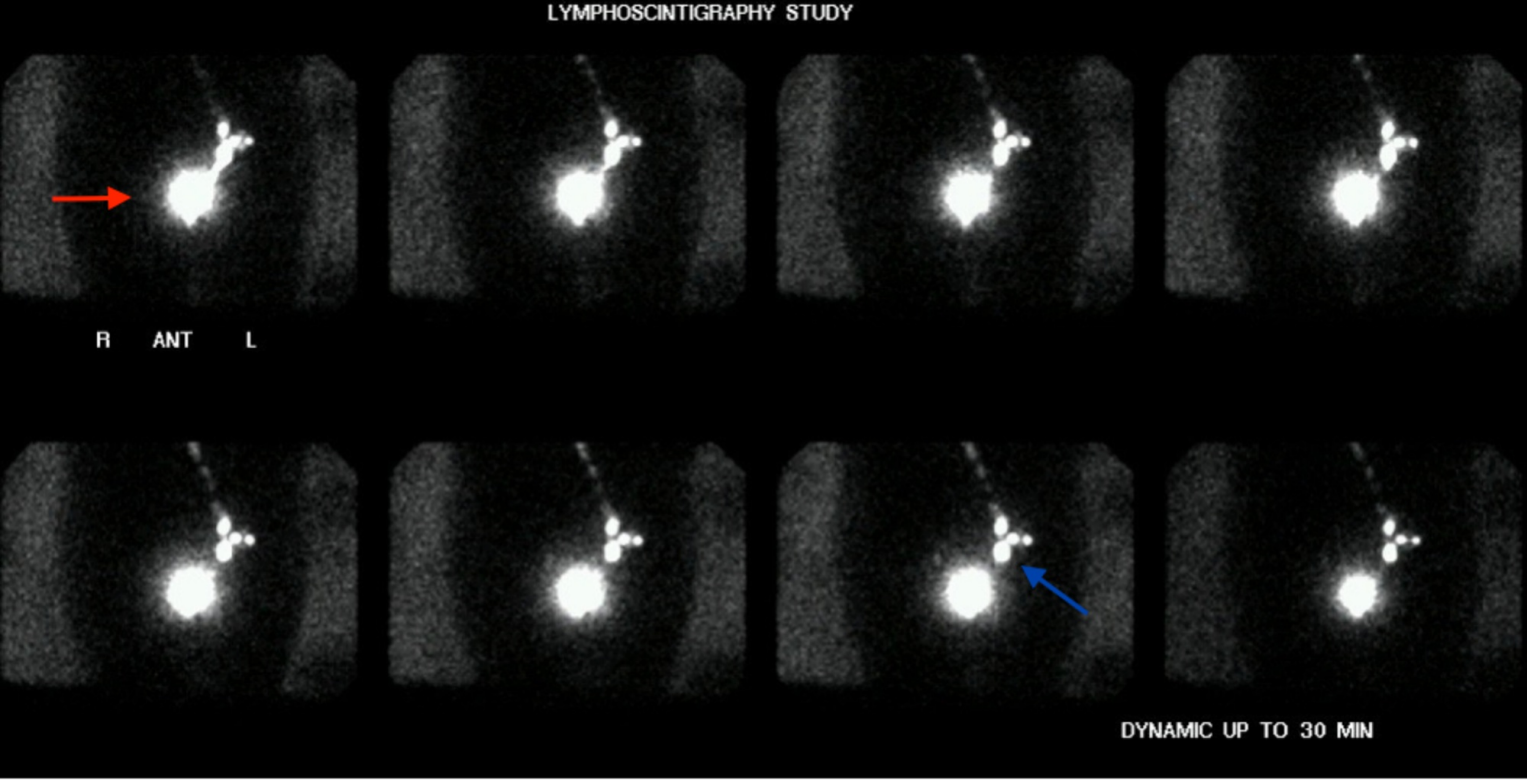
Lymphoscintigraphy study, anterior images Injection of technetium 99 m sulfur colloid at the site of melanoma lesion (red arrow). Dynamic anterior images demonstrate the filling of three lymph nodes in the left groin via a single lymphatic channel. The inferior node (blue arrow) is the SLN (sentinel lymph node); the superior nodes are secondary nodes.
